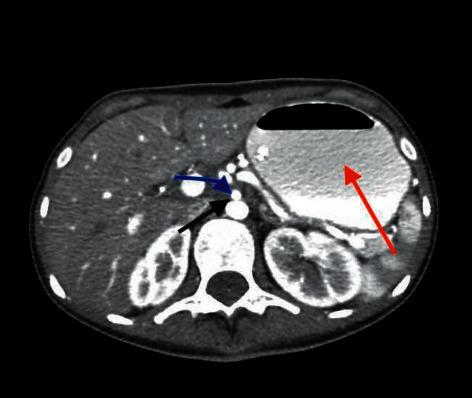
Axial CT abdomen showing compression of the third portion of the duodenum (black arrow) from the superior mesenteric artery (blue), with distention of the stomach (red arrow).
